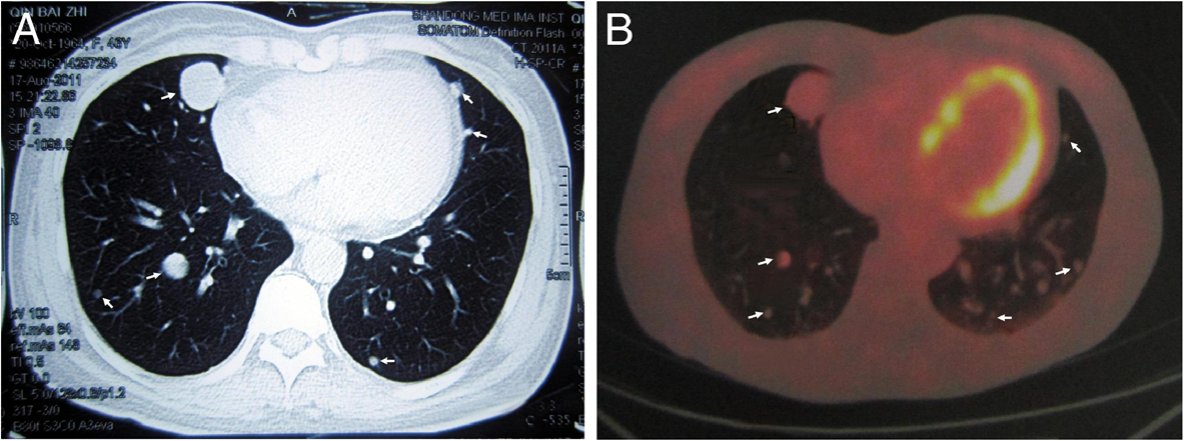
A Chest computerized tomography scan shows multiple nodules (arrows) in both lungs. B The PET scan shows no abnormal fluorodeoxyglucose uptake with suspicion of multiple nodules, and the SUV value was about 2.
